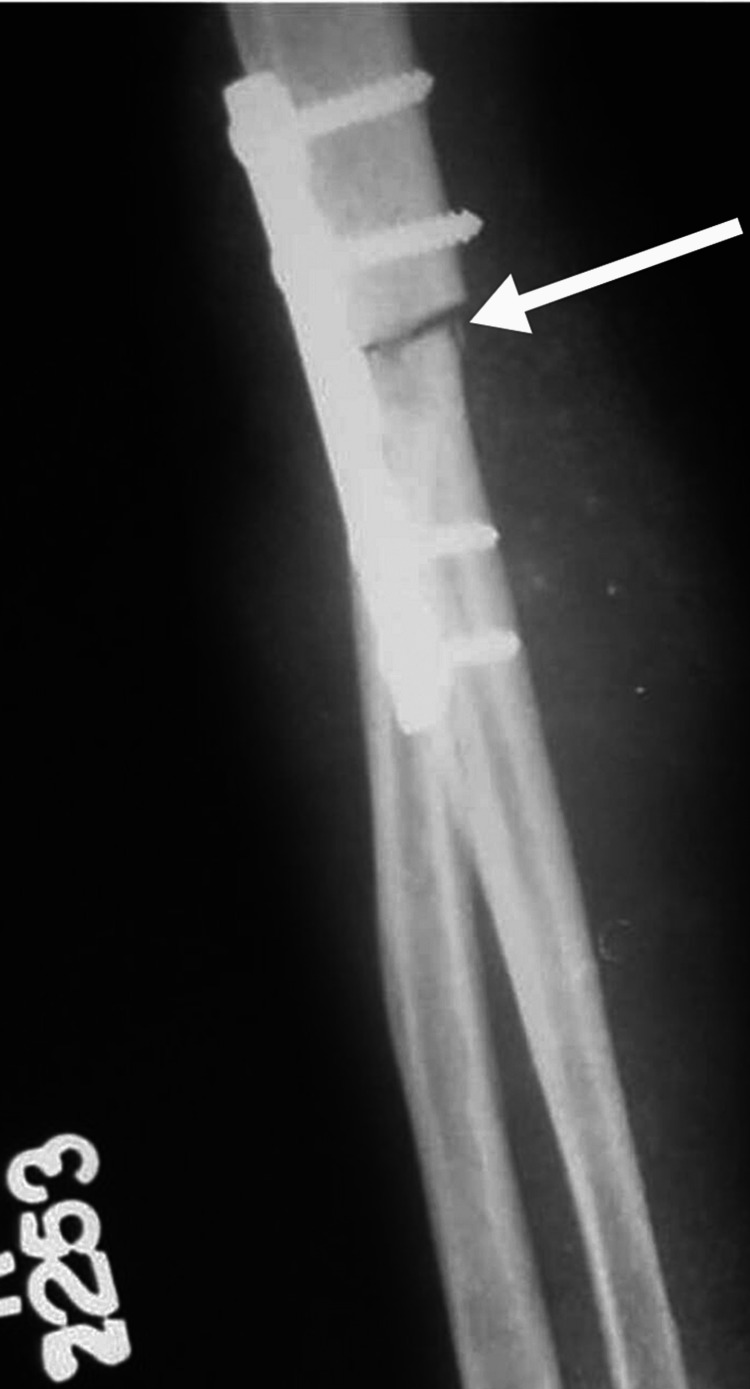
Postoperative radiograph showing humero-ulnar arthrodesis White arrow - hmero-ulnar arthrodesis using a plate
